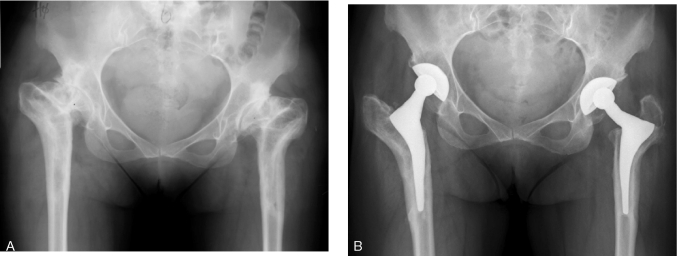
A. Bilateral hip dysplasia with valgus deformities after earlier subtrochanteric osteotomies. B. Radiograph after insertion of custom femoral stems bilaterally.
